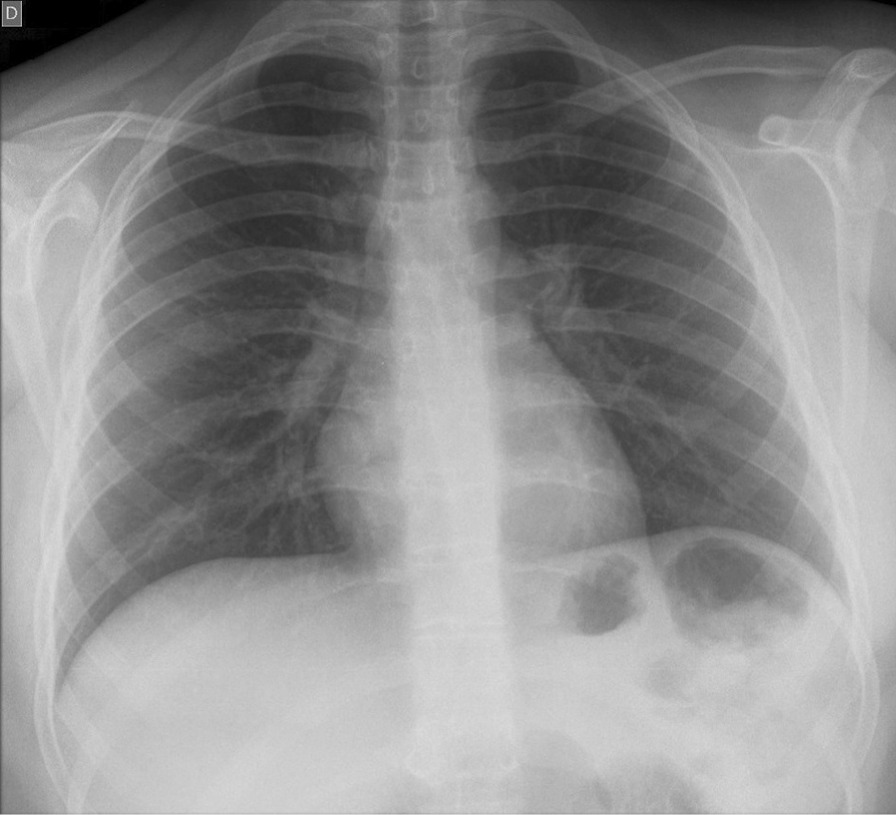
Chest radiograph in posteroanterior view. The radiological appearance of lung parenchyma was normal, which aided in ruling out sarcoidosis, a possible differential diagnosis
